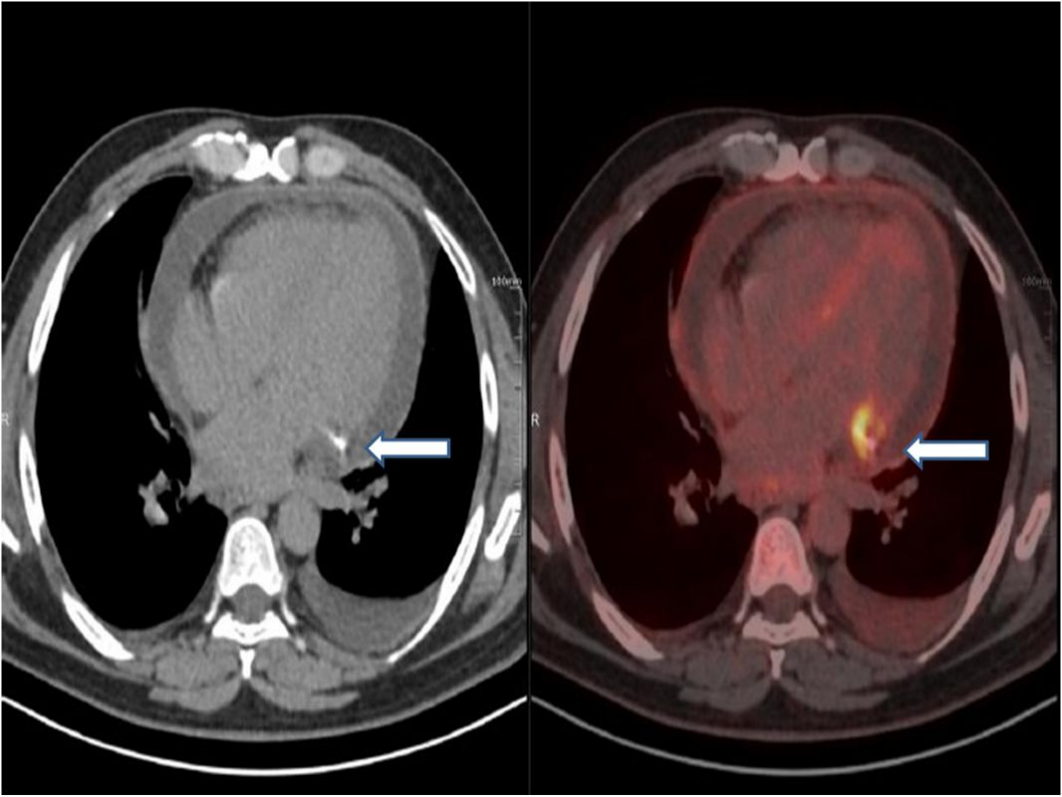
Positron emission tomography-CT imaging revealing elevated FDG activity surrounding the left circumflex artery stent in the left atrioventricular groove (arrow denotes), indicative of inflammation or infection at the stent site.
